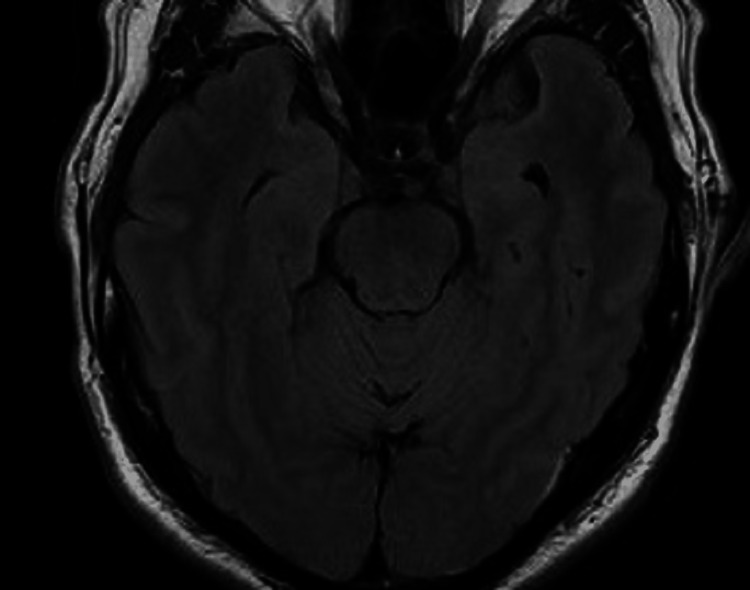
MRI fluid-attenuated inversion recovery (FLAIR) sequence 13 hours after the episode
